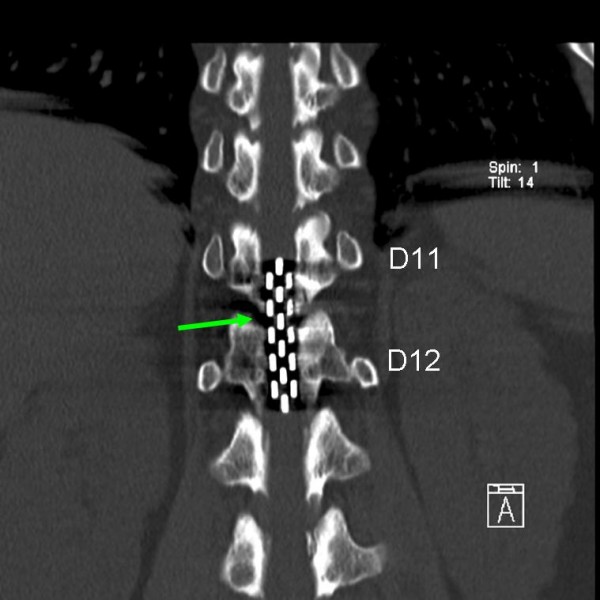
Coronal view of computed tomography scan of the lower dorsal spine. Green arrow points to Specify 565 electrode at levels D11 and D12.
